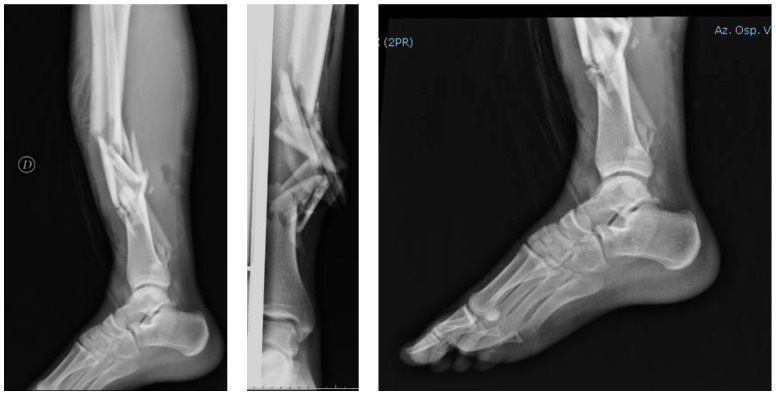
Pre-surgical emergency radiographs; anteroposterior (AP) and left lateral (LL) projections of the right leg and ankle.
